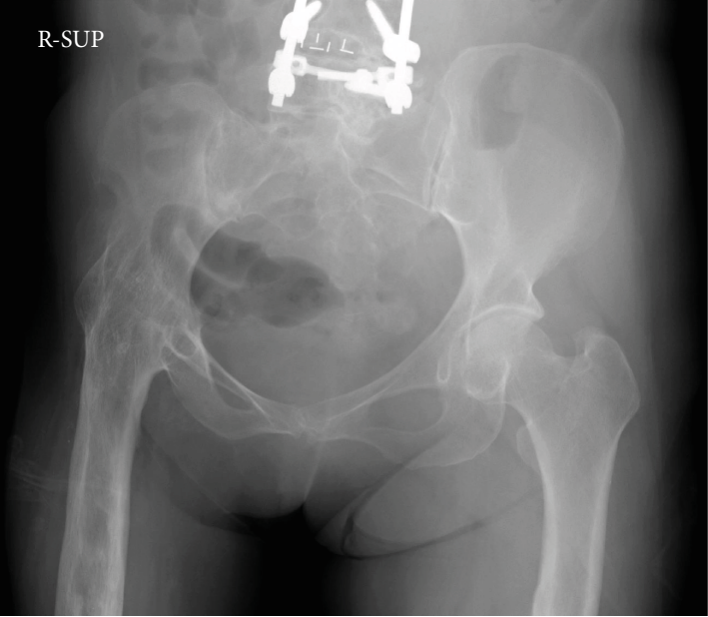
Radiography of the pelvis shows arthrodesis of the right hip joint and posterior lumbar interbody fusion.
